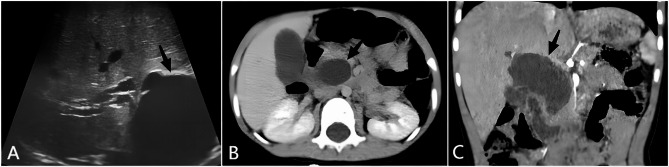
Routine imaging studies before surgery. A: Abdominal ultrasonography revealed cystic dilatation of the common bile duct measuring 6.3 × 3.7 × 3.1 cm (arrow); B: CT transverse section view of the CDC surrounded by the pancreas (arrow); C: CT coronal section view of the CDC (arrow)
